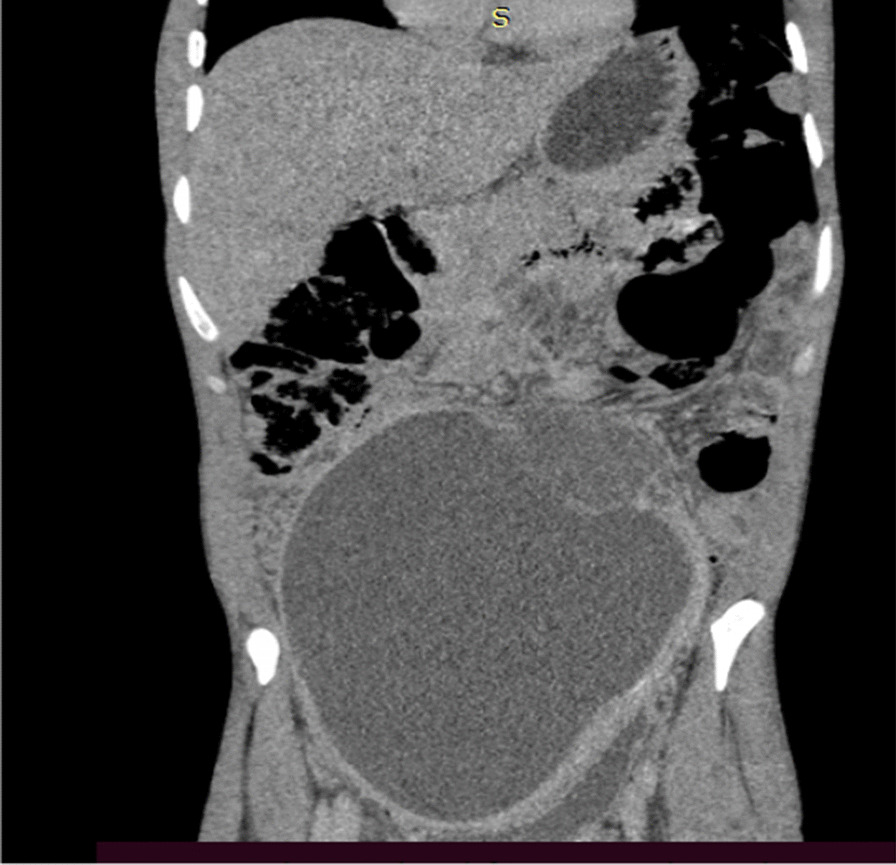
CT scan showing a predominantly cystic lesion that had an enhancing wall with heterogeneous mural soft tissue
